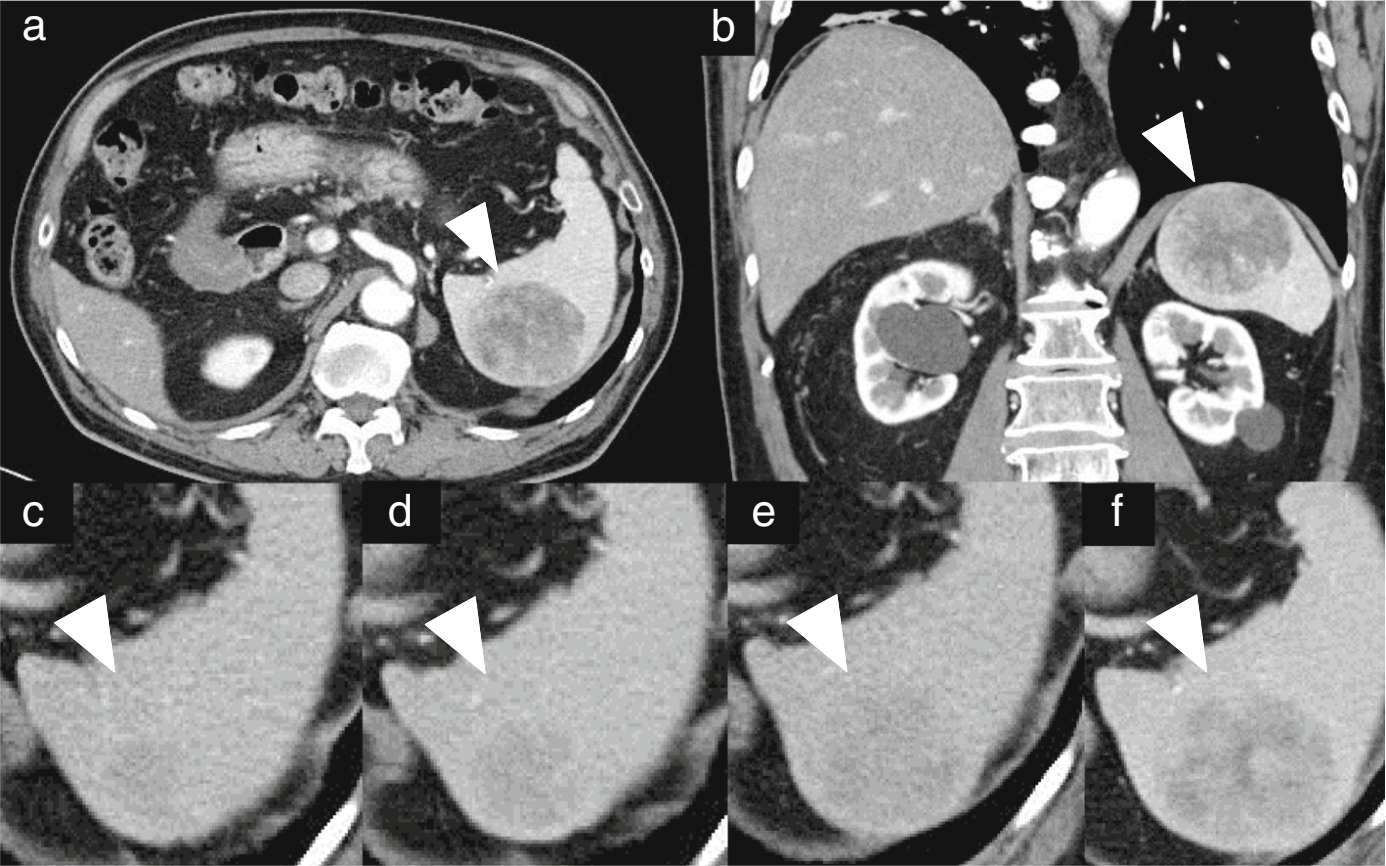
a and b: Computed tomography imaging shows a sharply circumscribed 50 mm tumor with slightly decreased uptake and a heterogeneous appearance in the spleen. c-f: A nodule appeared five years after thymectomy and enlarged gradually for three years thereafter. d: Six years after thymectomy. e: Seven years after thymectomy. f: Eight years after thymectomy. Arrows in all panels point to the metastatic lesion in the spleen
